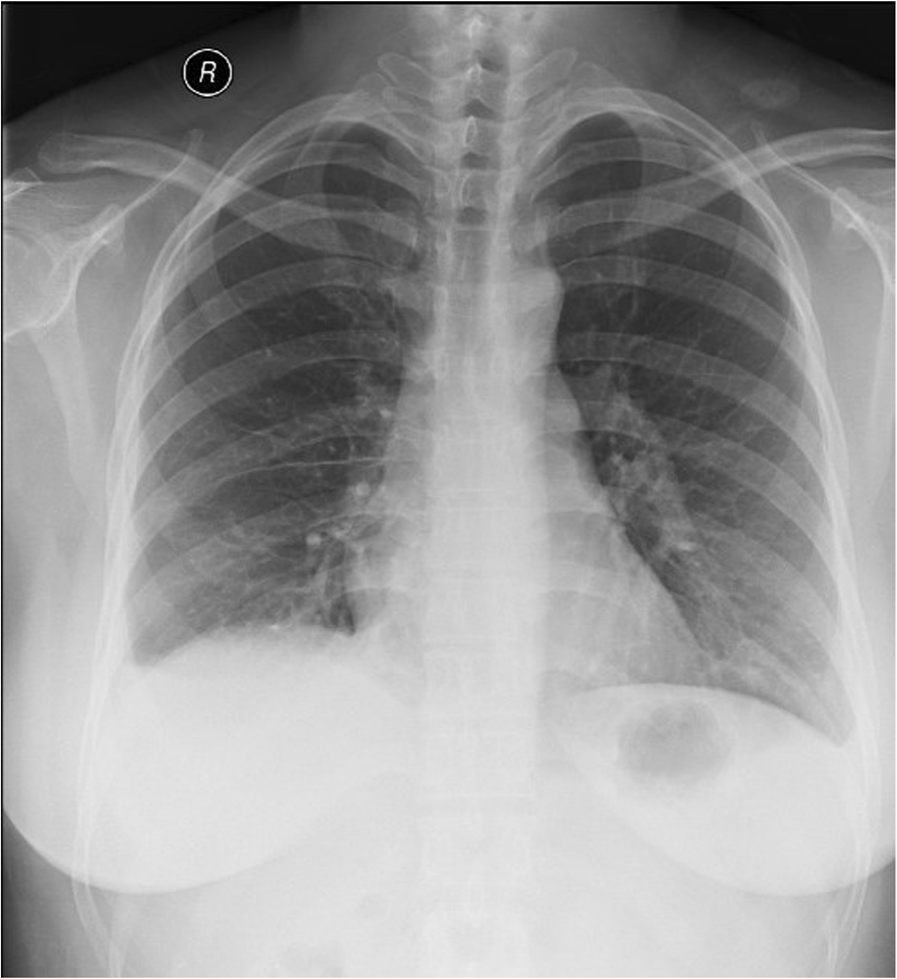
Chest X-ray on the first postoperative day
